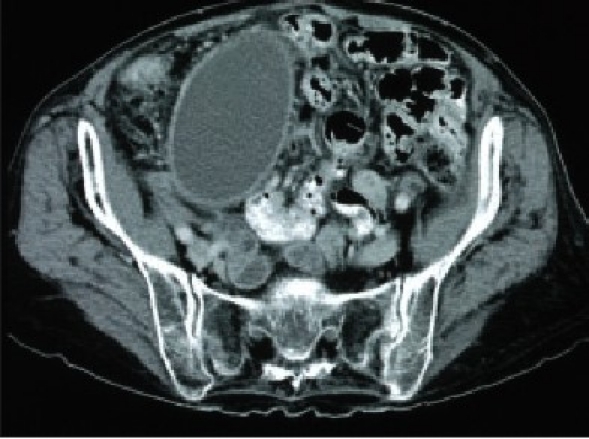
Computerized tomography (CT) scan demonstrating massive gallbladder extending to the pelvic brim
